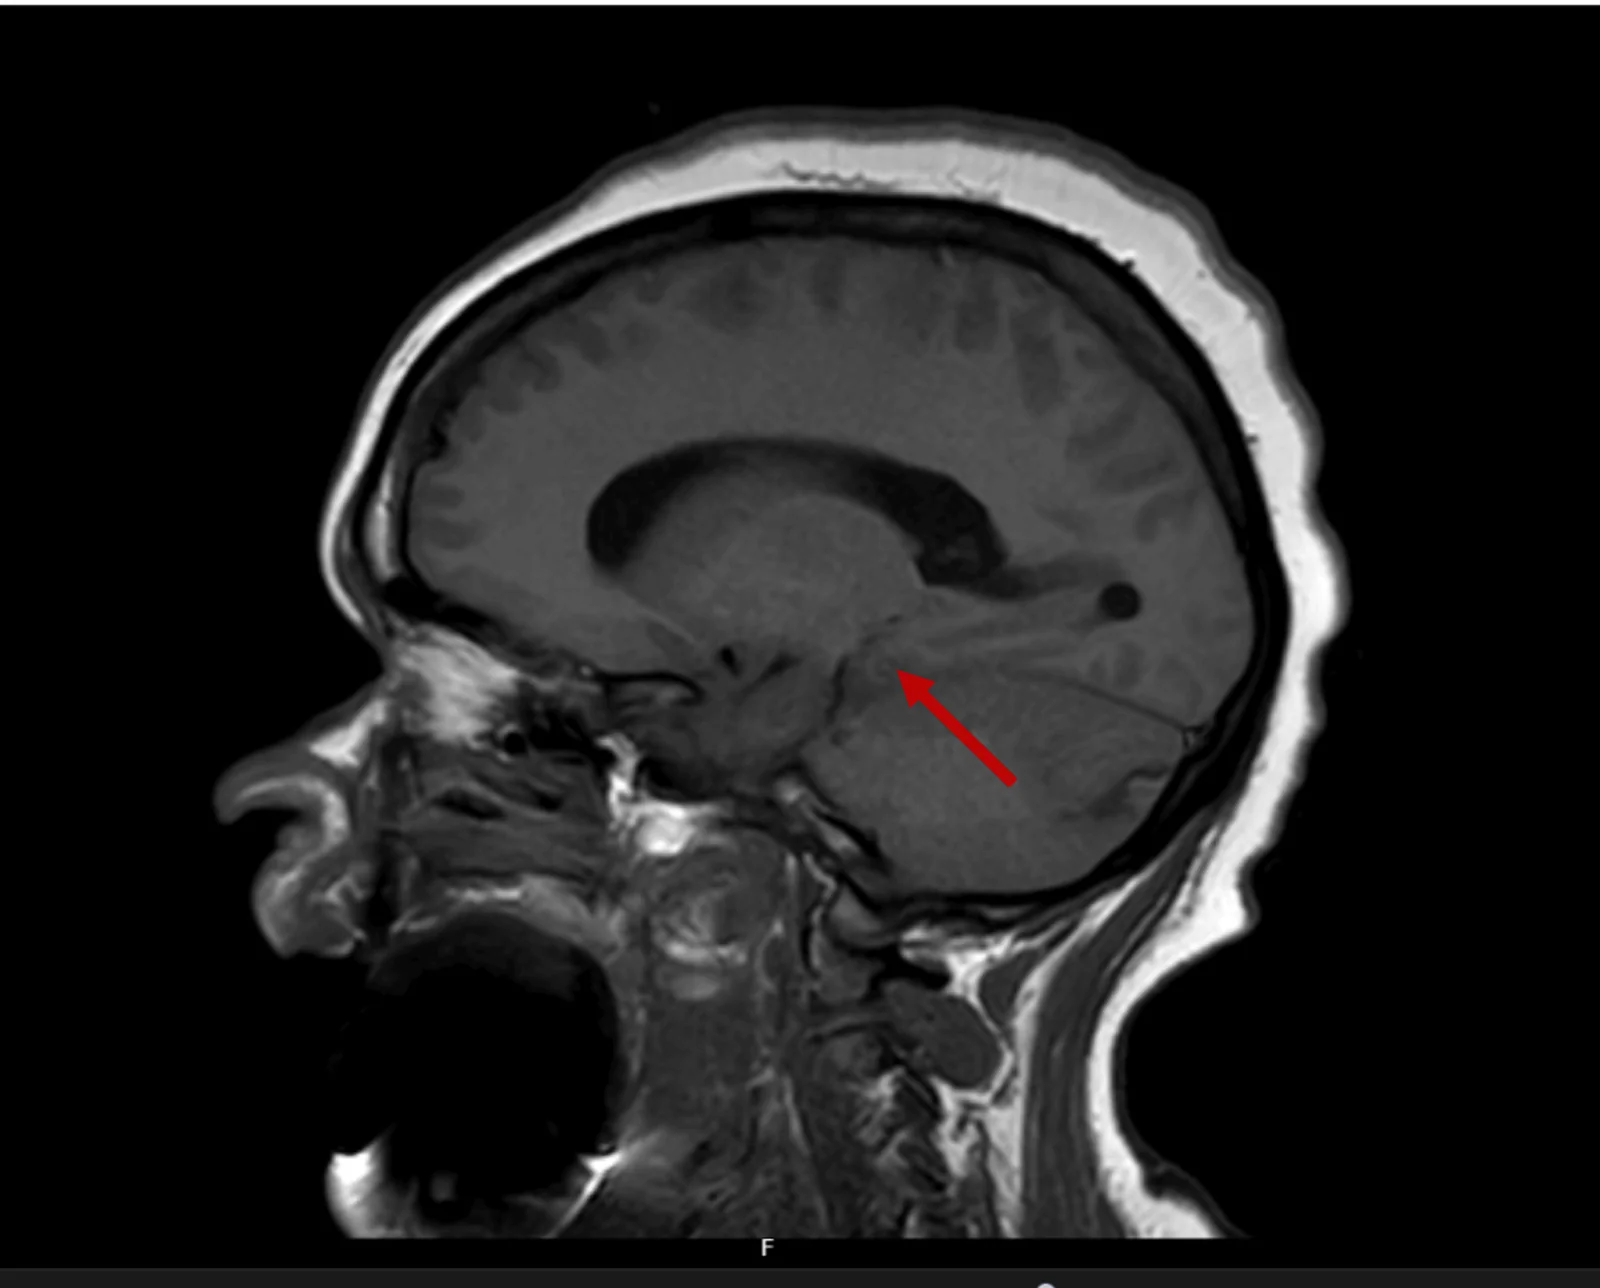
Sagittal T1-weighted MRI Posterior fossa showing transtentorial and tonsillar herniation (arrow).
